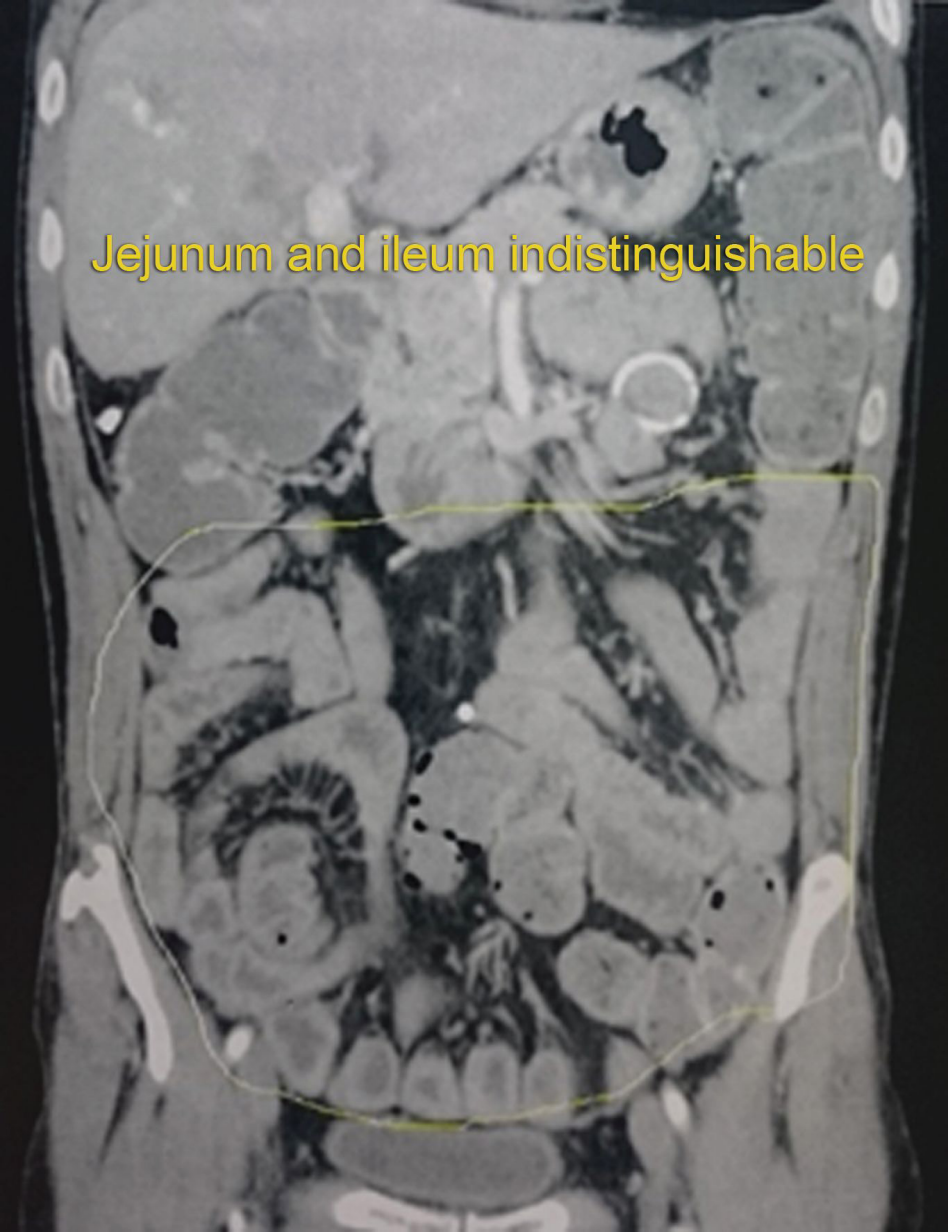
Computed tomography with contrast showing edema of small intestine loops.
